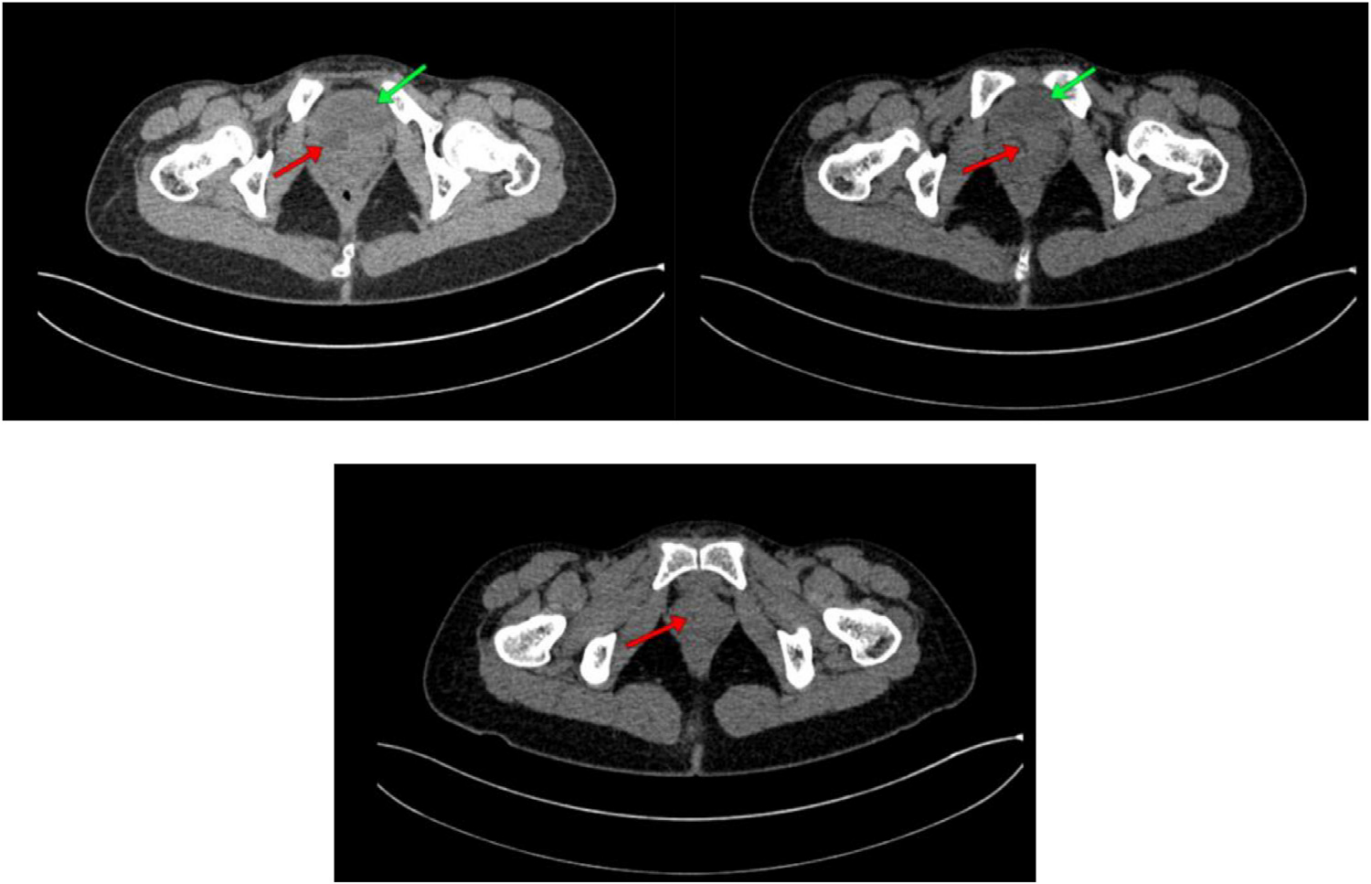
Noncontrast abdominal CT scan. Green arrow: bladder; red arrow: dilated and tortuous ureter still running below the urinary bladder.
